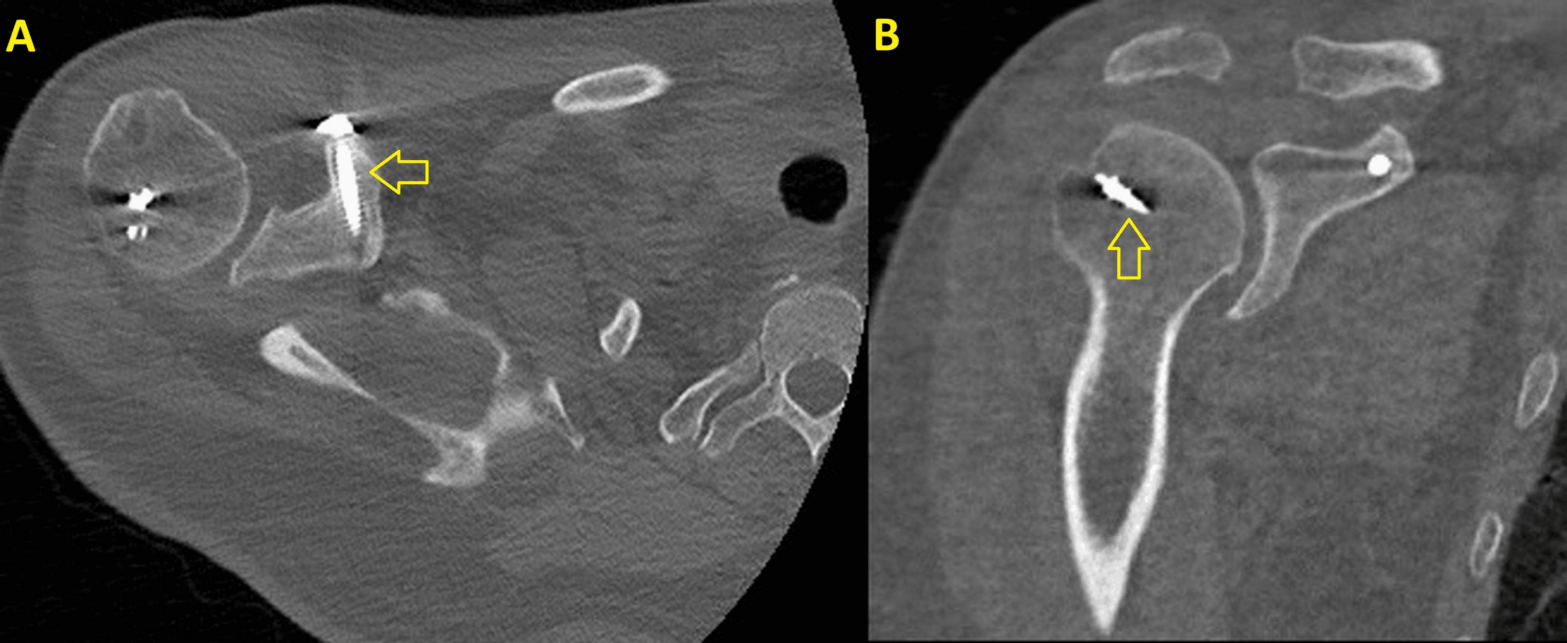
CT at six months postoperatively. (A) Transverse view: The yellow arrow points to cortical lag screw in the coracoid process. (B) Coronal view: The yellow arrow indicates one of the anchors used for rotator cuff repair.
